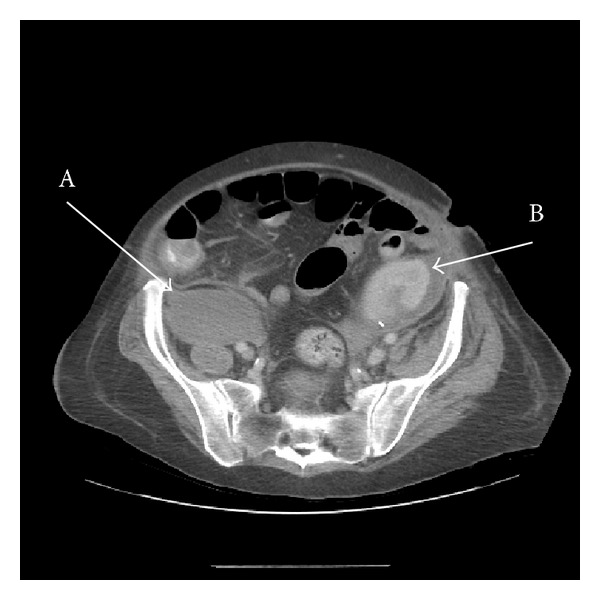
CT scan with contrast showing bilateral psoas haematomata with the right extending to a much lower level within an intact psoas muscle compartment (A: right iliopsoas haematoma; B: transplant kidney).
